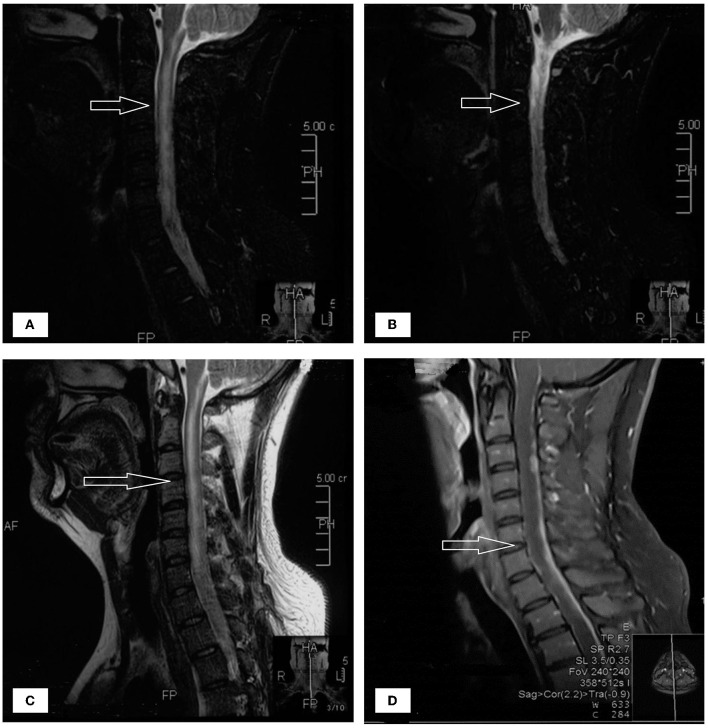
(A–D) The patient's spinal MRI showed multiple abnormal signals in the central canal of spinal cord, as shown by the arrow.
